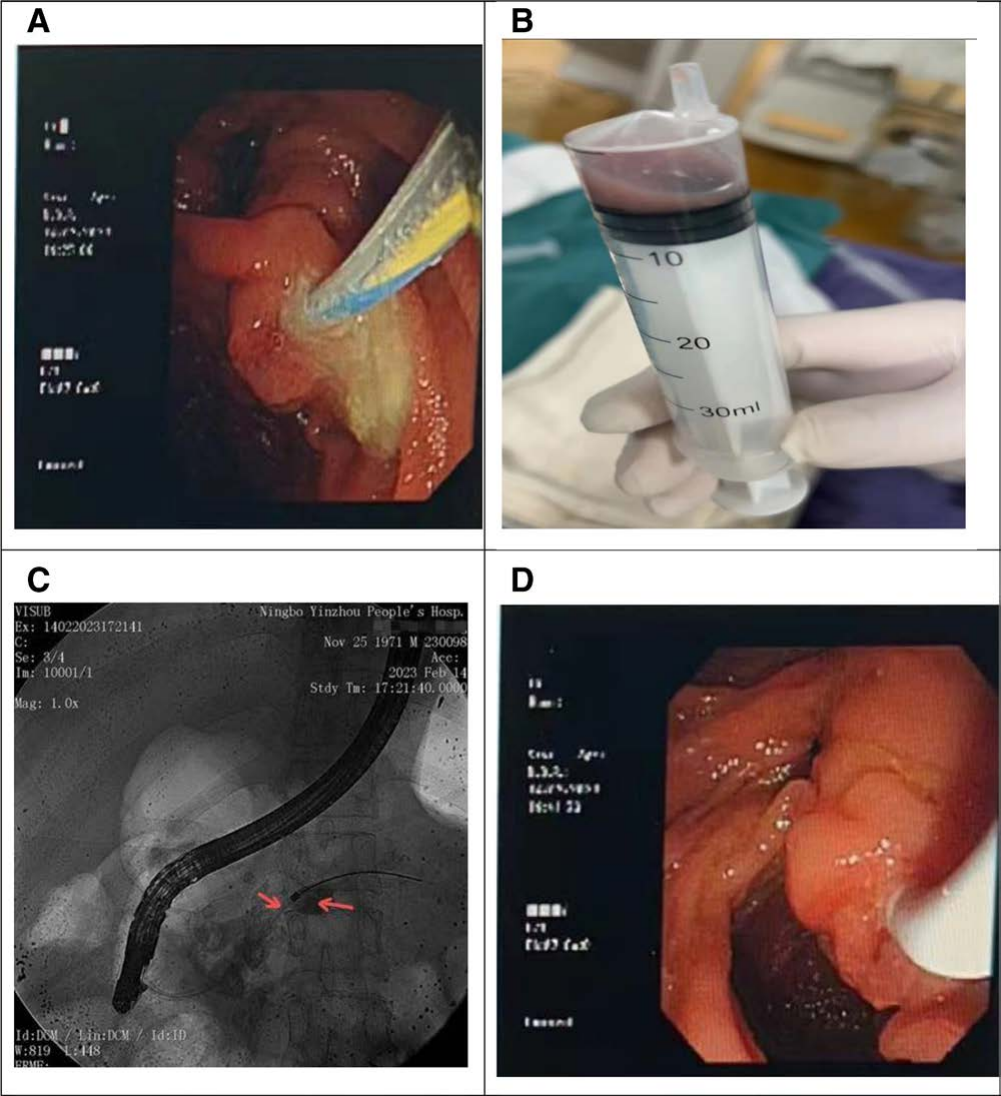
Operations and findings in ERCP. (A) The yellowish-white turbid liquid at the duodenal papilla. (B) 5 mL of purulent fluid with a strong foul odor. (C) X-ray findings: the arrows indicate the location of the stenosis (left) and the dilated pancreatic duct (right). (D) A 7Fr nasopancreatic tube is placed in the body and tail of the pancreas. ERCP = endoscopic retrograde cholangiopancreatography.
